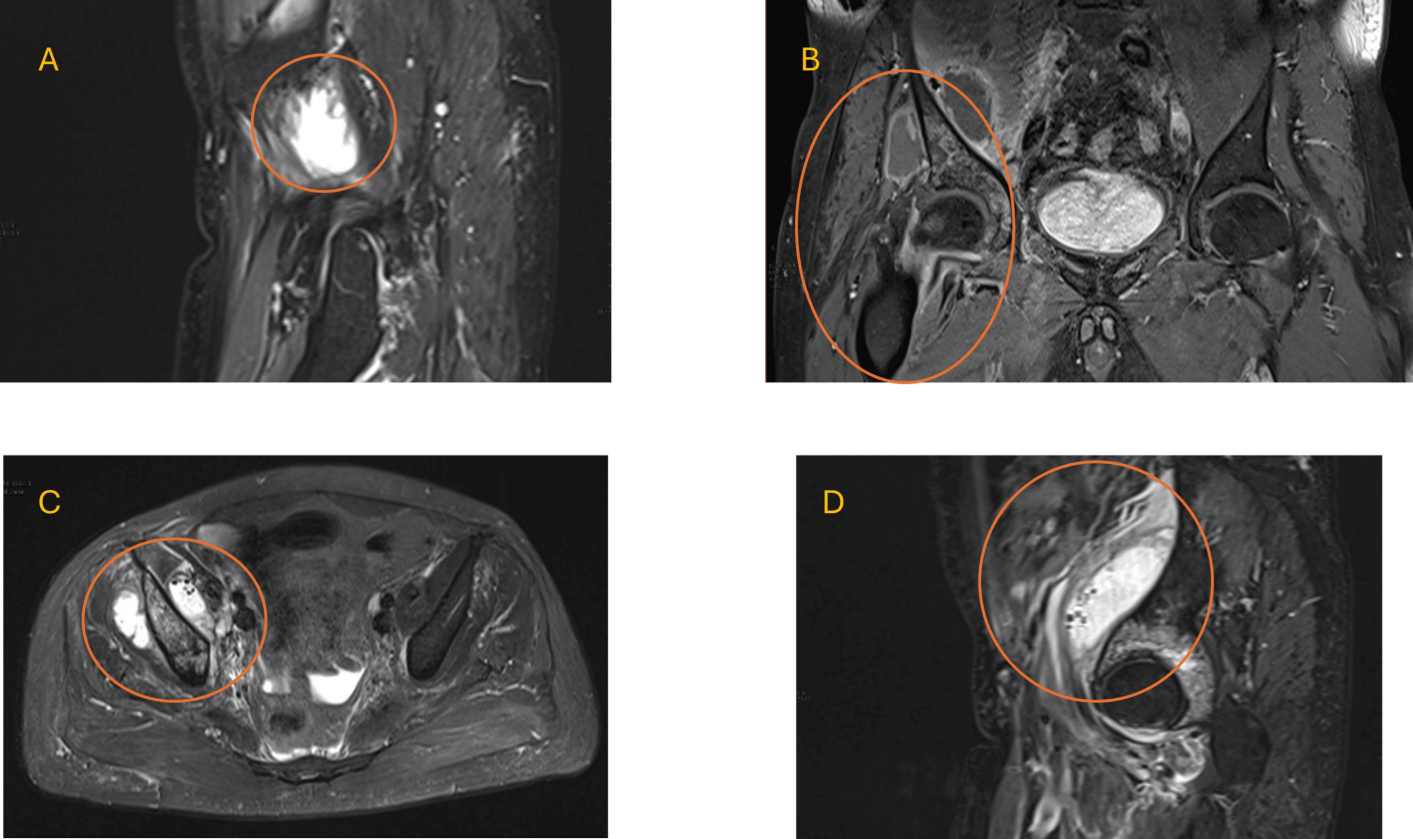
Abdomen and pelvis MRI with contrast. Pelvic MRI demonstrating collections in the iliac and gluteus minimus muscles with associated edema and reactive osteitis in adjacent bones. Panel A: Sagittal T2-weighted MRI showing the right iliac muscle, with ring-enhancing collection. The abnormality is hyperintense in the T2-weighted image. Panel B: Coronal T2-weighted MRI demonstrating a 3×5.8×8.6 cm ring-enhancing collection and a 4.8×3.8×3.3 cm with minimal edema involving the right iliac muscle and the right gluteus minimus. Note the irregular borders and heterogeneous signal intensity. Panel C: Axial T2-weighted MRI depicting ring-enhancing collection. The lesion is located in the right iliac and the right gluteus minimus muscles. Panel D: Another sagittal T2-weighted view highlighting an additional view of the abnormality noted in Panel A. The abnormal structure is seen in proximity to the right iliac muscle.
